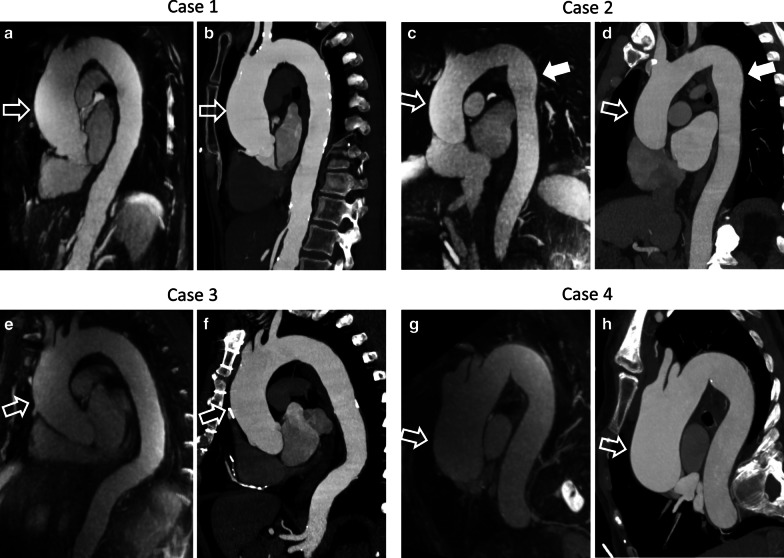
Representative case examples demonstrating comparison between CMRA and computed tomography angiography (CTA). Representative CMRA (a, c, e and g) and CTA (b, d, f and h) maximum intensity projection images displayed as 5 mm thick slabs are shown in the candy cane view of the aorta. Case 1 (a CMRA and b CTA) features a 68-year-old woman with ascending aortic dilatation (maximum diameter of 49.2 mm, open arrows). Case 2 (c CMRA and d CTA) shows a 73-year-old man with dilatation affecting both the ascending (open arrows) and the descending aorta (solid arrows) with maximum diameters of 44.0 mm and 35.8 mm, respectively. Case 3 (e CMRA and f CTA) presents a 64-year-old man with dilatation predominantly in the ascending aorta reaching a maximum of 45.3 mm (open arrows). The descending aorta has a borderline maximum diameter of 30.7 mm. Case 4 (g CMRA and h CTA) shows a 77-year-old woman with a tortuous thoracic aorta, likely accentuated by scoliosis. The maximum diameter of the ascending aorta (open arrows) is 52.6 mm
